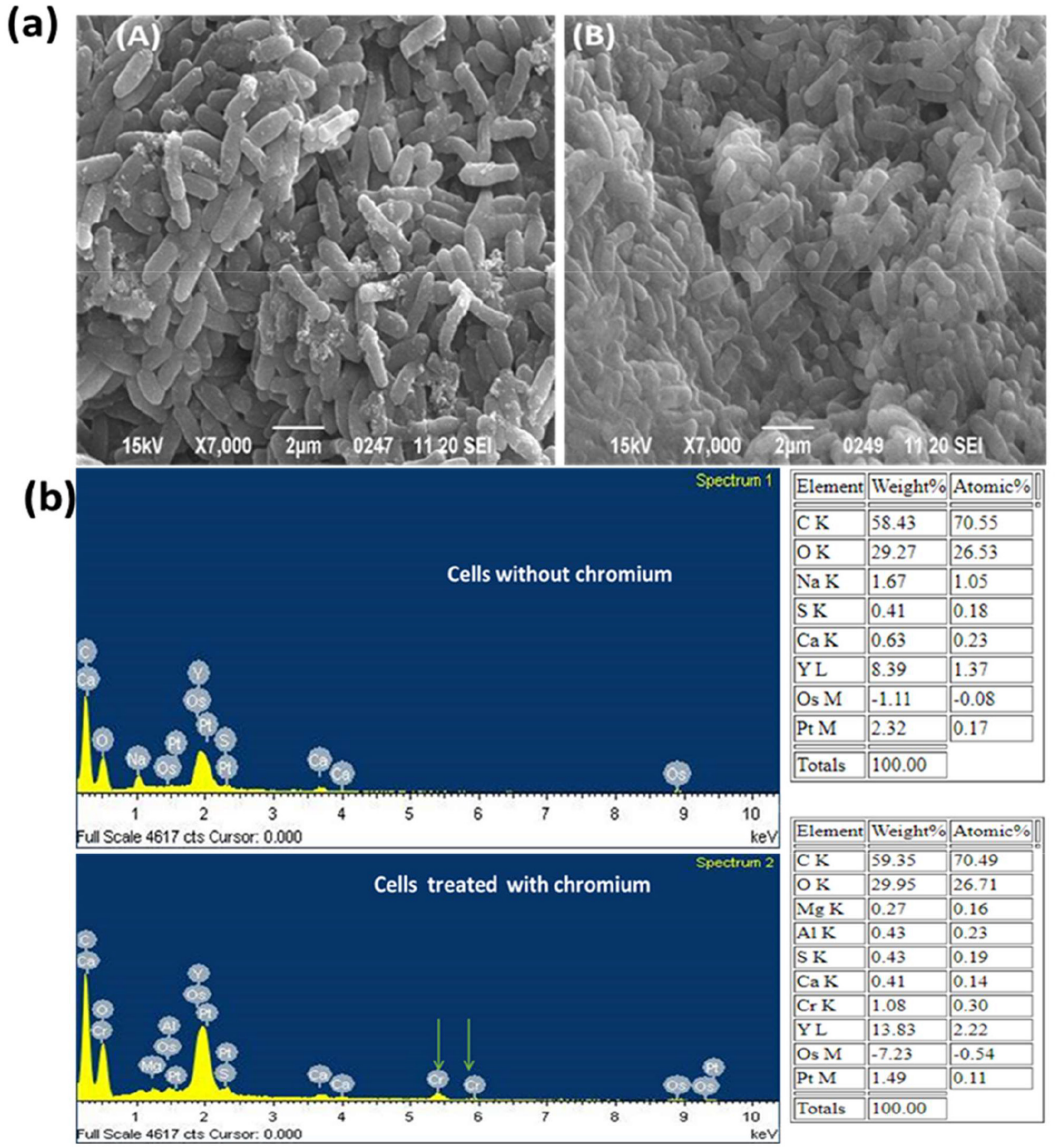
(a) SEM analysis of chromium untreated (A) and chromium treated cells (B) of *B. licheniformis* strain KNP; (b) Energy Dispersive X-ray (EDX) analysis of chromium untreated and chromium treated cells *of B. licheniformis* strain KNP.
